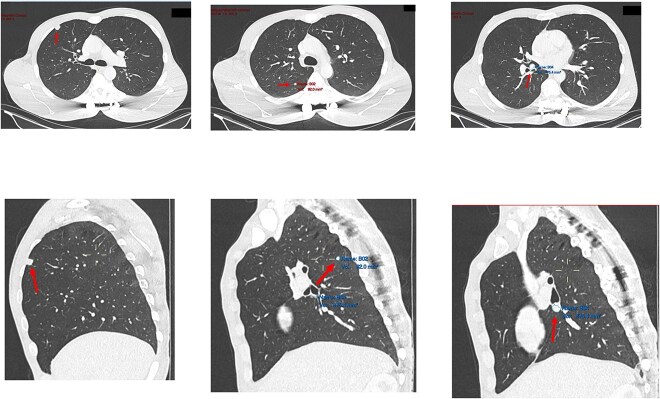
Transverse and saggital views of the three nodules identified on an investigative CT scan.
